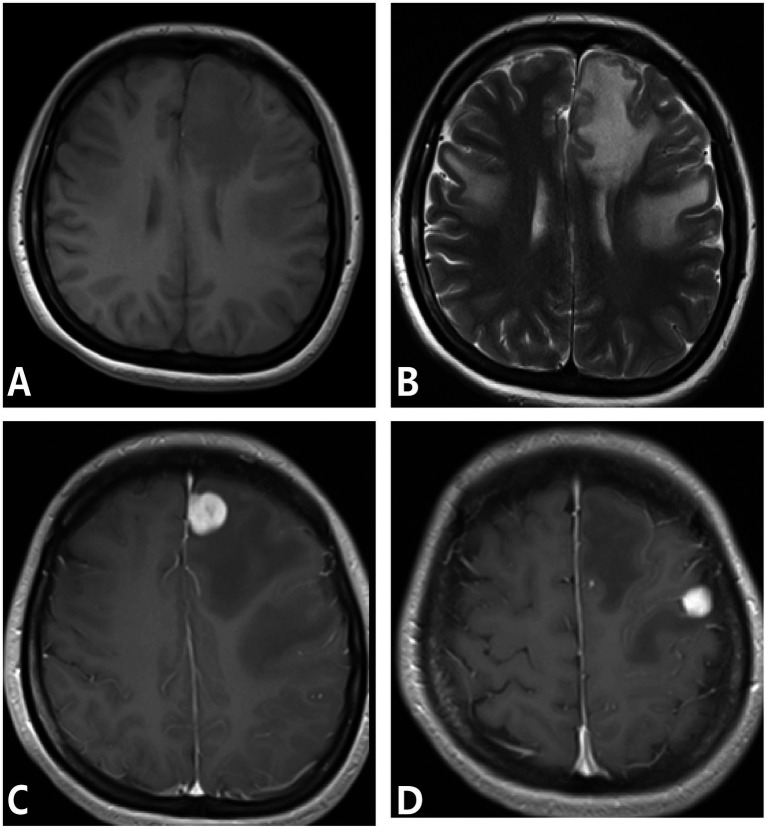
(A–D) Syphilis gumma type neurosyphilis, a 39-year-old male presented headache for 7 months, with paroxysmal right facial twitching for 1 month. MRI showed multiple lesions in the left frontal lobe surrounded by an edematous zone with marked enhancement. Panel (A) (T1WI): shows isointense mass-like lesions; panel (B) (T2WI): depicts hyperintense nodular lesions (arrow) with peripheral edema; Panel (C) (T1WI-CE): shows contrast-enhanced nodular lesions with surrounding edema; panel (D) (T1WI-CE): demonstrates marked nodular enhancement and adjacent meningeal enhancement.
